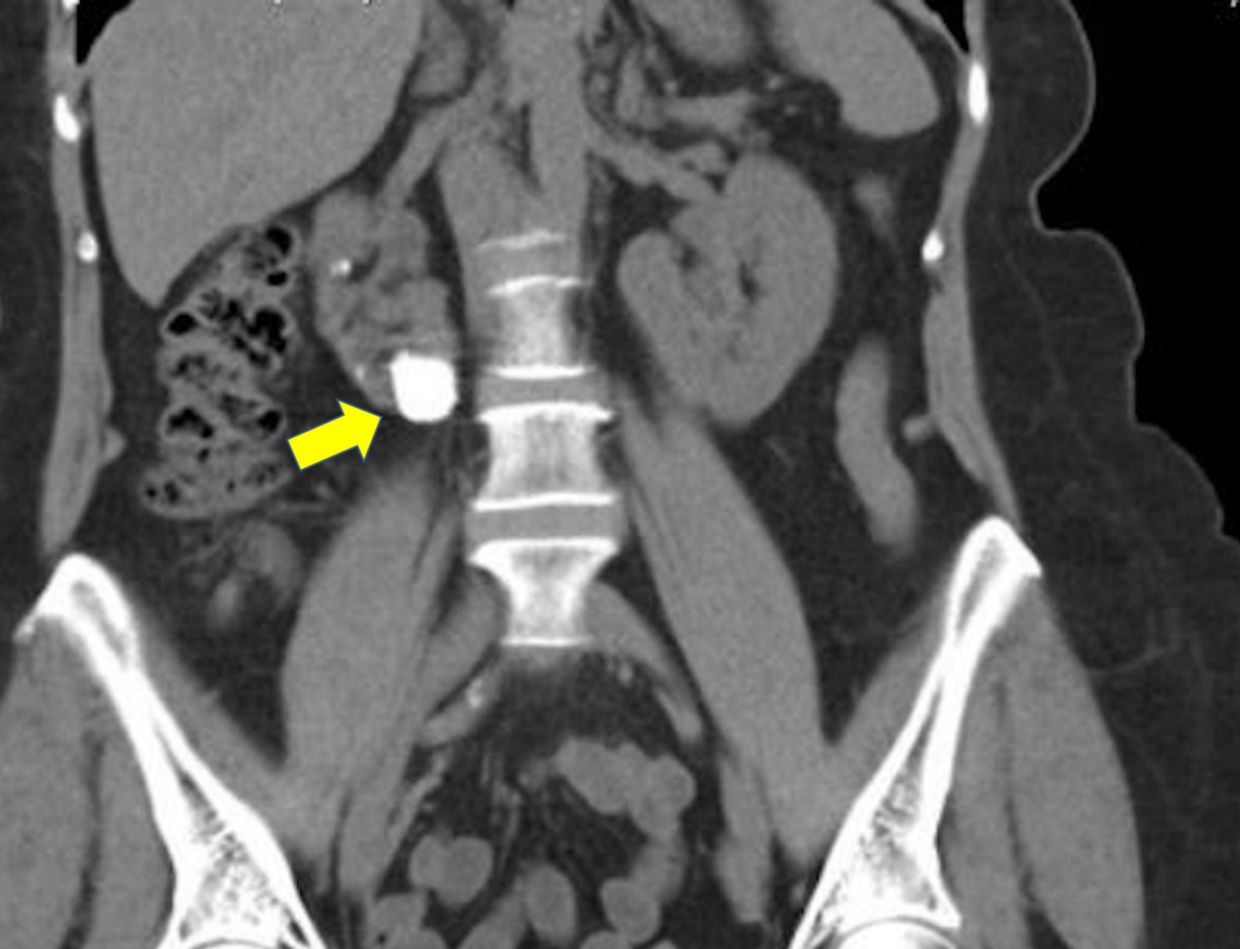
A coronal view of non-contrast CT scan showing the lower pole calculus
